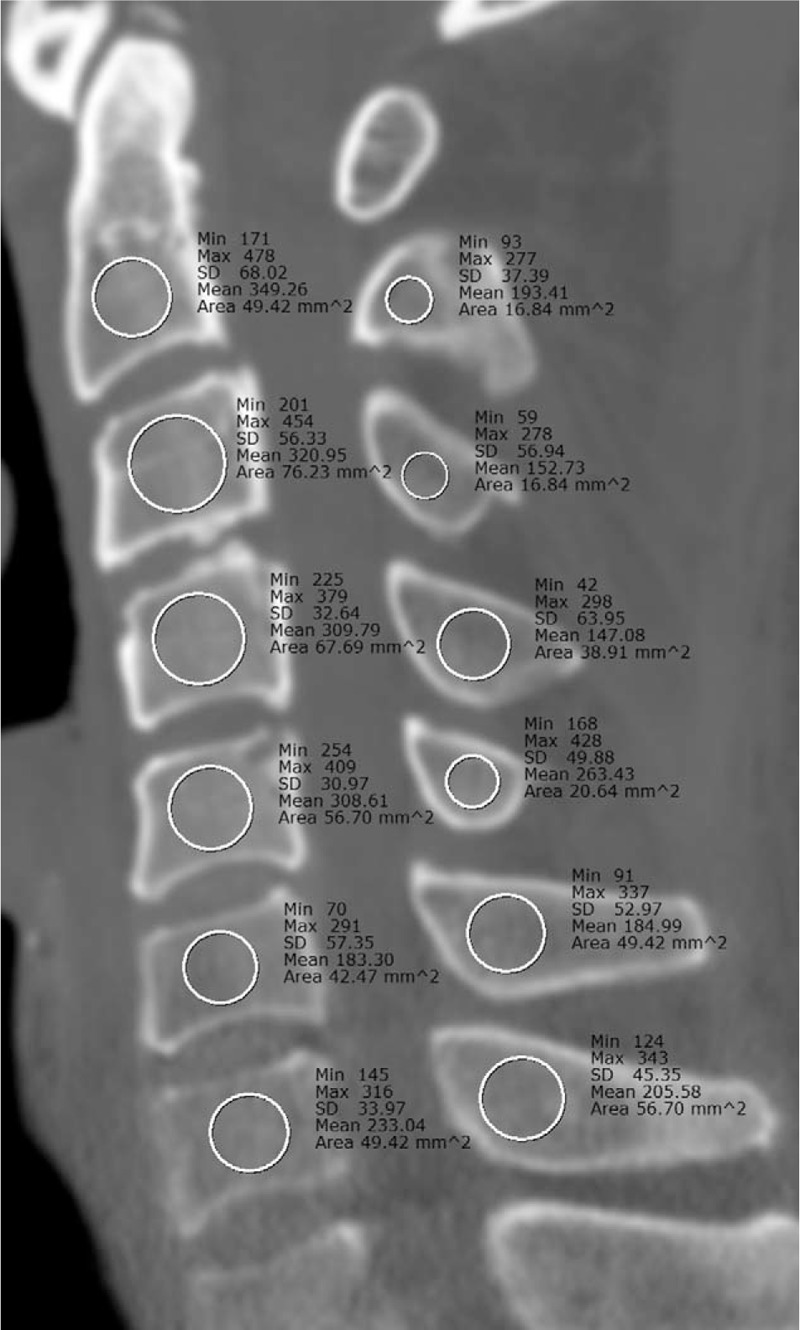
CT number measurement method. For comparison of the mean CT number between the MRCT and conventional CT images, the mean CT number (HU) at the midline of the vertebral body and spinous process at each cervical spine (six segments of C2–C7, a total of 12 measurements/pt) is measured using sagittal MRCT and conventional CT images. In the vertebral body, the circular ROI was manually positioned in the largest area of the bone not including the area of the cortical bone. HU = hounsfield unit, MRCT = magnetic resonance-generated synthetic computed tomography, ROI = region of interest.
